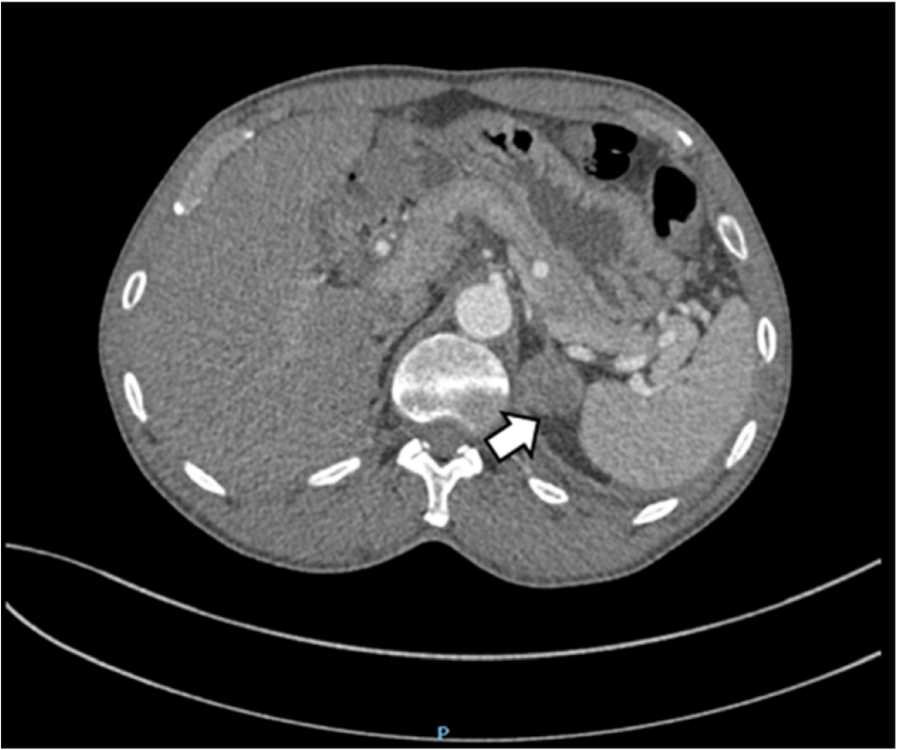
Contrast computed tomography scan shows mass in the left adrenal gland. Arrow: left adrenal tumor
